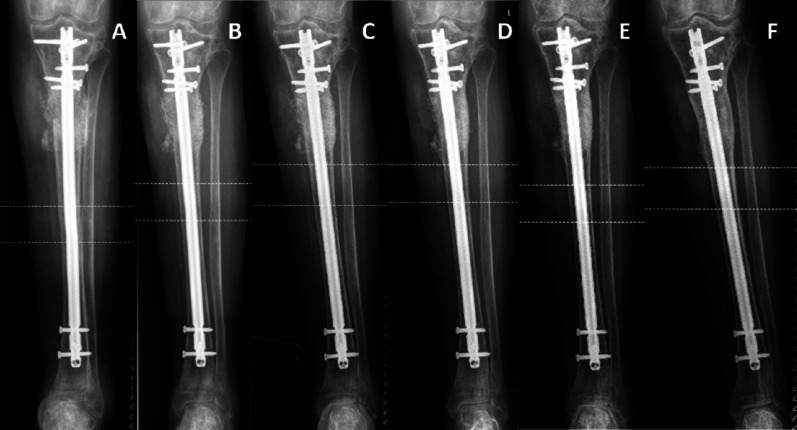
Example of a non-union during a 5-year follow-up; Post-operative X-rays after A six weeks B twelve weeks C six months D one year E two years (F) five years
